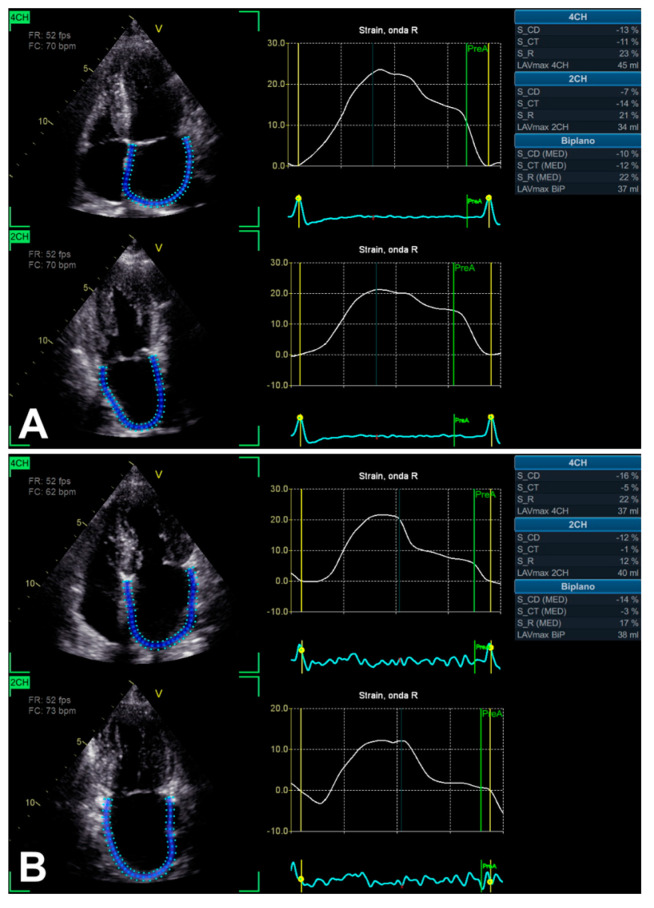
An example of LA function evaluation by LA strain technique, in the presence of SAS, assessed from 2D apical four-chamber and two-chamber views. In two asymptomatic patients with relatively similar LV EF (66% and 60%), notably, biplane LA reservoir peak atrial longitudinal strain (PALS) is different: >20% (A) and <20% (B), respectively.
